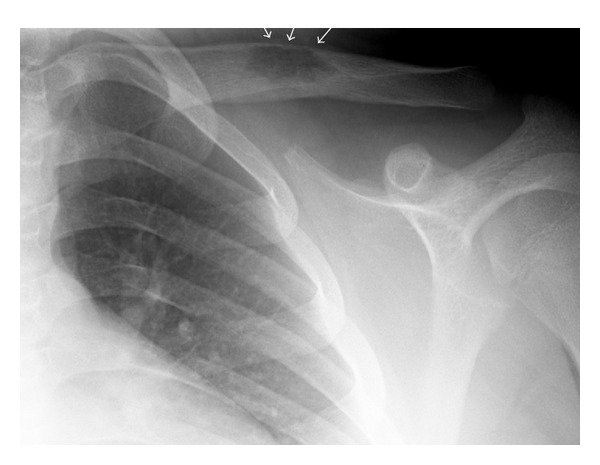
An AP radiograph of the left clavicle in a 13-year-old boy shows a mid-clavicular lytic lesion and subtle periosteal reaction superiorly (arrows). No internal matrix, surrounding sclerosis or soft tissue mass is seen.
